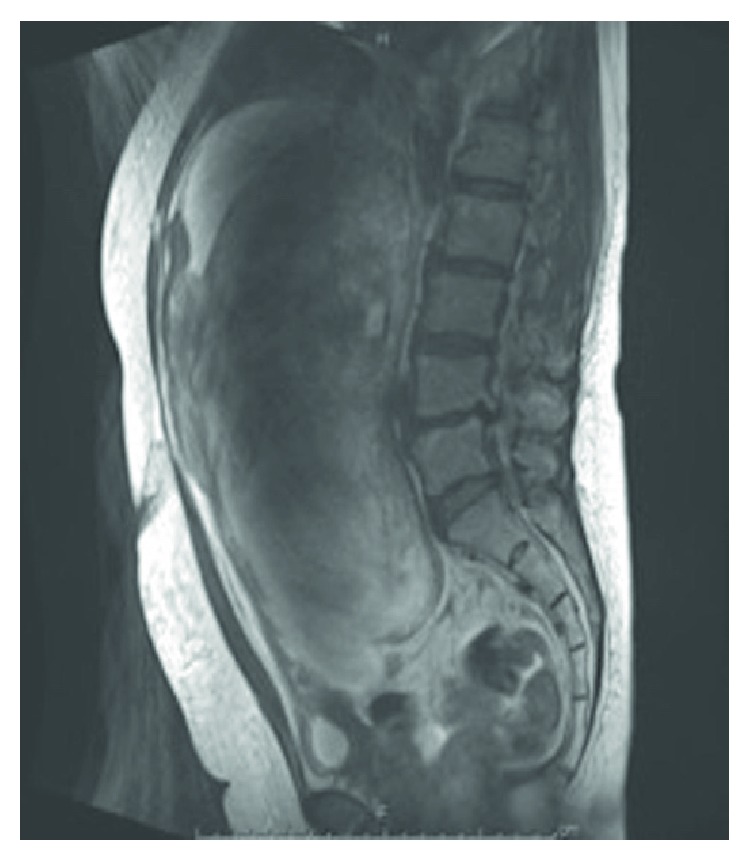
T2-weighted magnetic resonance imaging.
